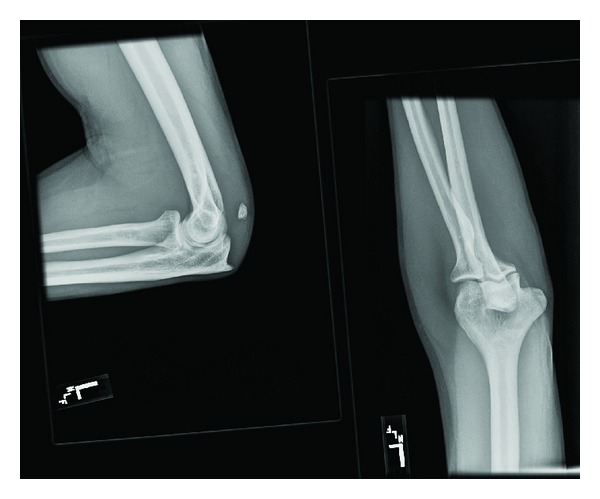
Plain radiograph, left elbow with avulsion fracture.
